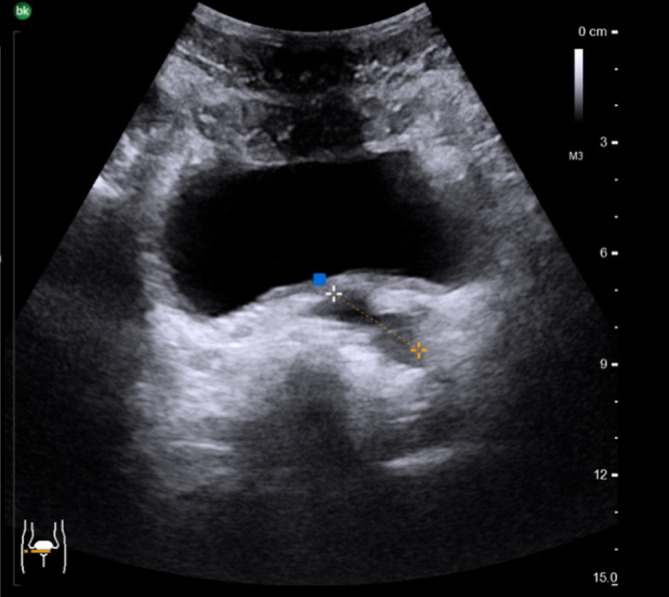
Sonographiebefund von transvesikal mit prominenter linker Samenblase dorsal der Harnblase
